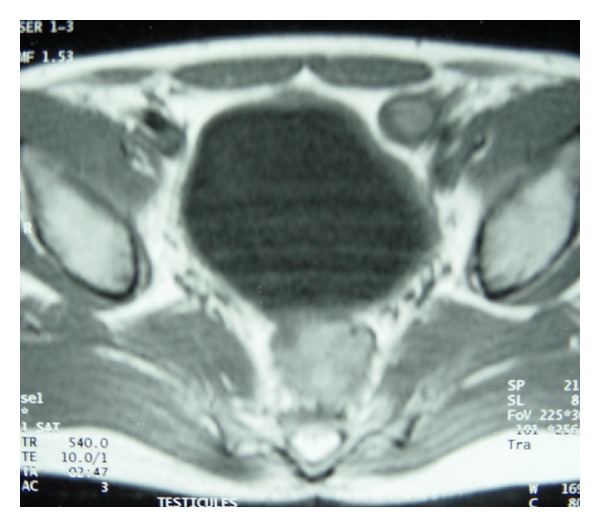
T1-weighted magnetic resonance image of the pelvis showing ectopic position of the left testis in front of the left commoun iliac vessels.
